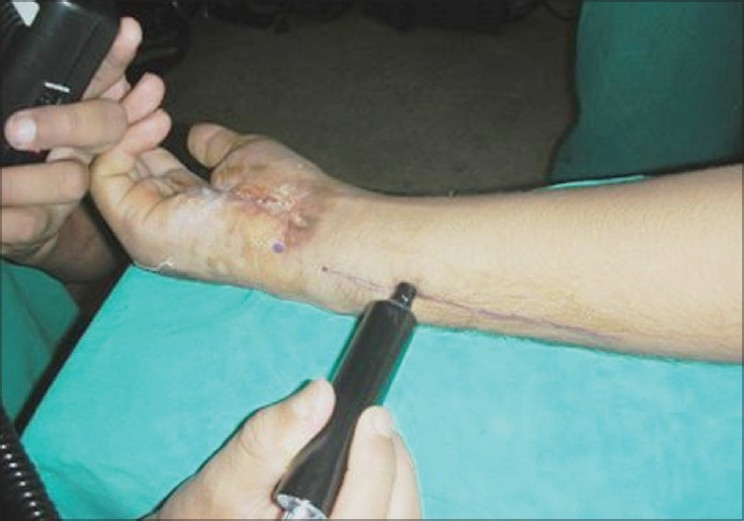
Localization of dorsal ulnar artery with doppler
